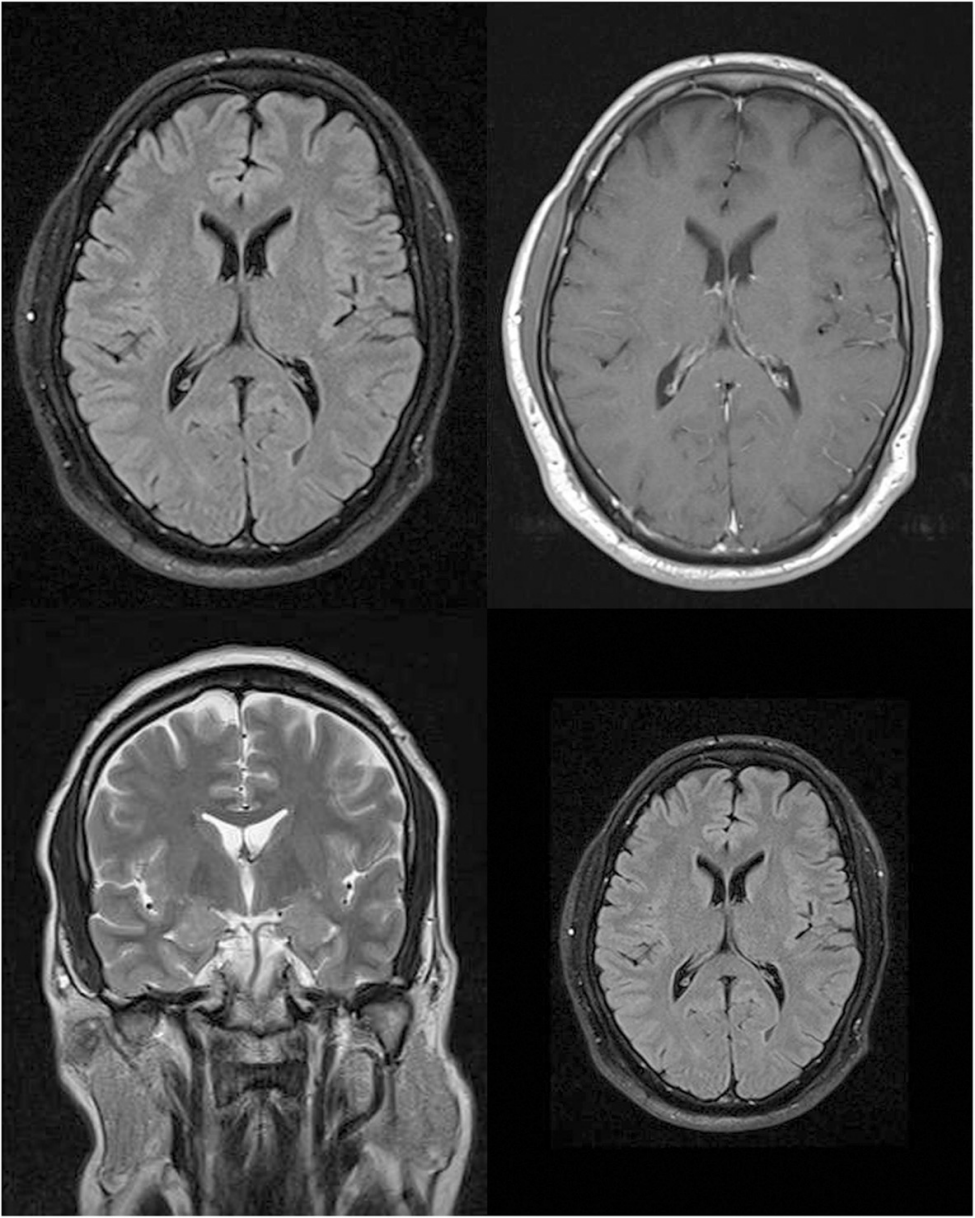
Brain magnetic resonance imaging images.
